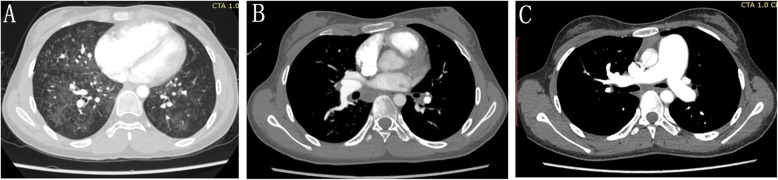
Representative chest CTA image obtained in a *EIF2AK4* mutation PVOD patient. Chest CTA axial section revealed ground-glass opacities and interlobular septal thickening (a), enlarged mediastinal lymph nodes (b), and a dilated pulmonary main trunk (c)
